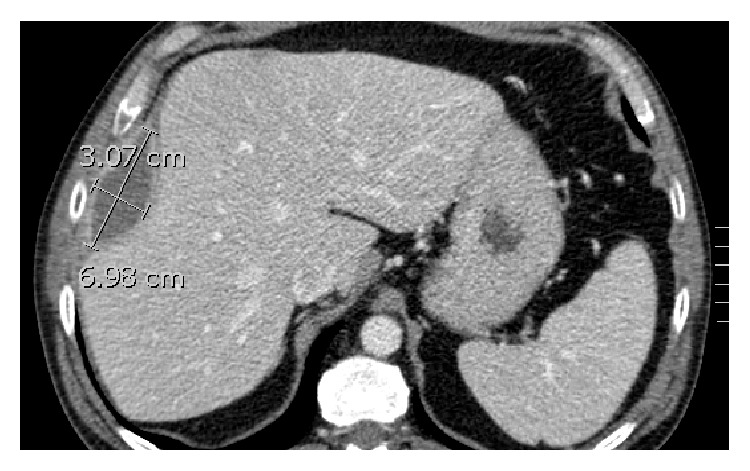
Gallstone-associated perihepatic abscess on preoperative computed tomography measuring 6.98 cm × 3.07 cm.
